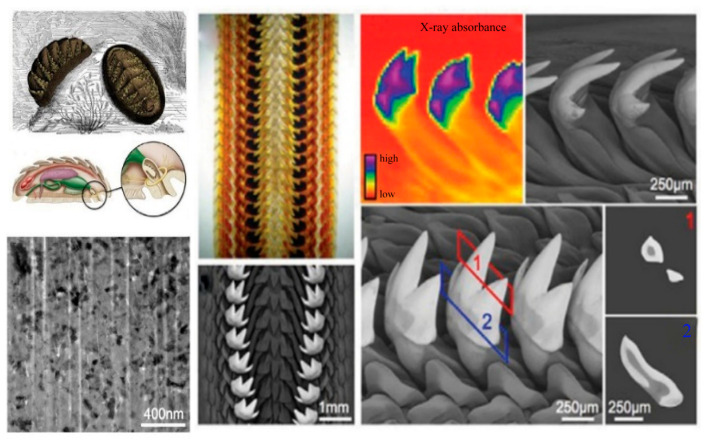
Morphological features of the chiton radula and synchrotron X-ray transmission map [27].
